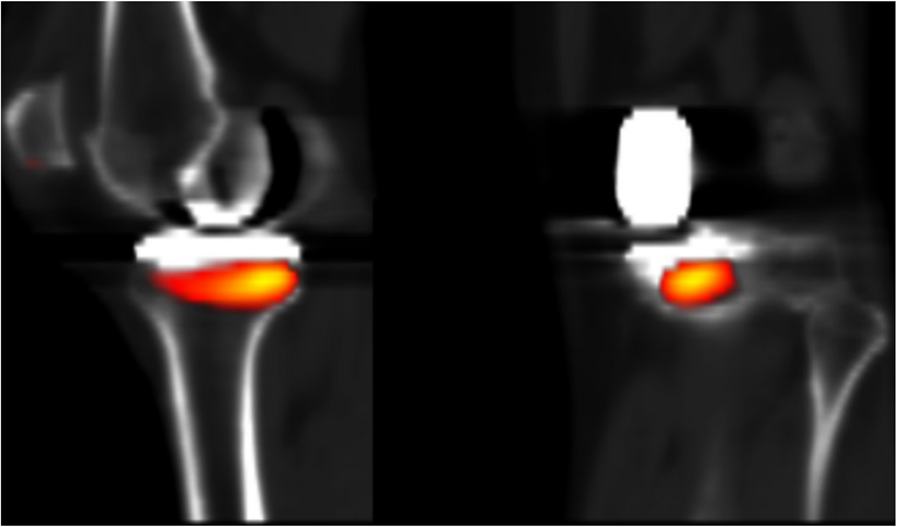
Average increased SPECT activity overlaid onto reference CT. Legend: Left: sagittal view, Right: frontal view. Intensity ranging from red (low activity) to yellow (high activity)
